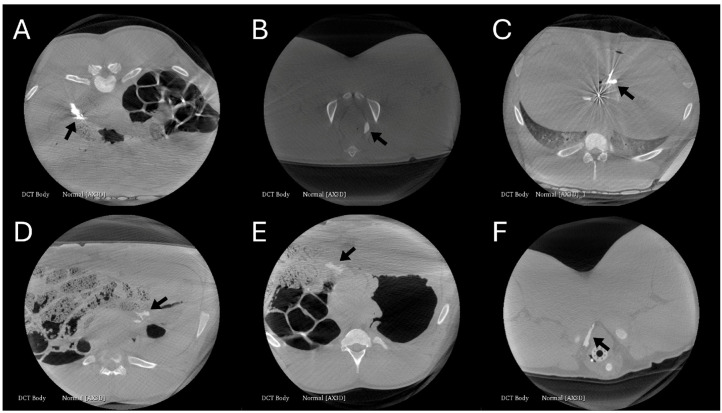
Arrows point at the injected contrast bolus showing relative visibility on CBCT. Insert (A) is 15% *v/v* (55.5 mg/mL), (B,C) are 10% *v/v* (37 mg/mL), and (D–F) are 5% *v/v* (18.5 mg/mL) Isovue-370 concentrations.
